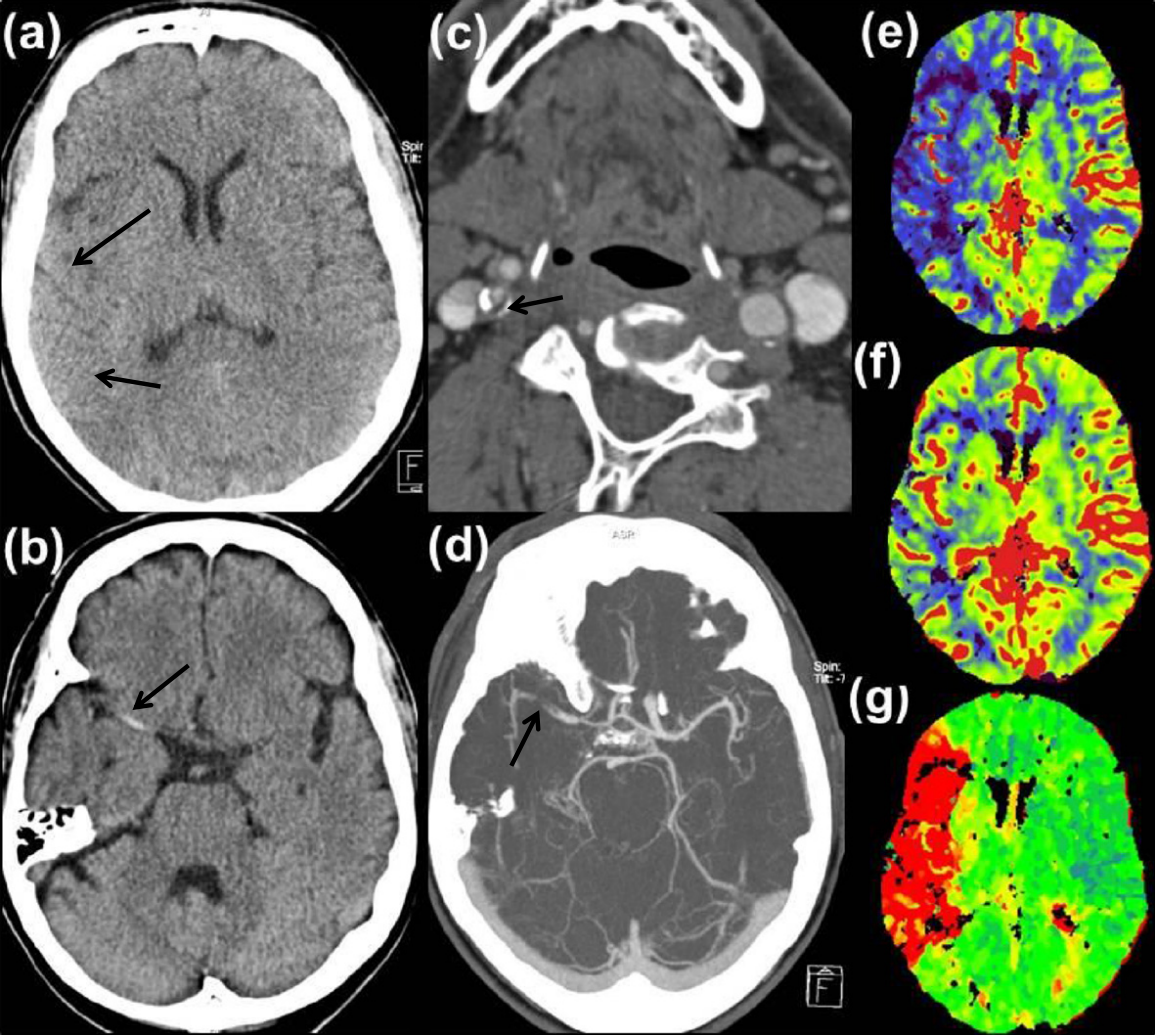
Early signs of ischemia in native cranial computed tomography scan with effacement of sulci and loss of the insular ribbon in right middle cerebral artery territory (a) and hyperdense right middle cerebral artery sign (b). High-grade calcified stenosis and thrombotic material in right internal carotid artery (c). Computed tomography angiography displaying a fresh thrombus in right middle cerebral artery (d). Computed tomography perfusion imaging with a clear perfusion deficit (red area of time-to-peak (g) and a significant mismatch of cerebral blood flow (e) to cerebral blood volume (f).
